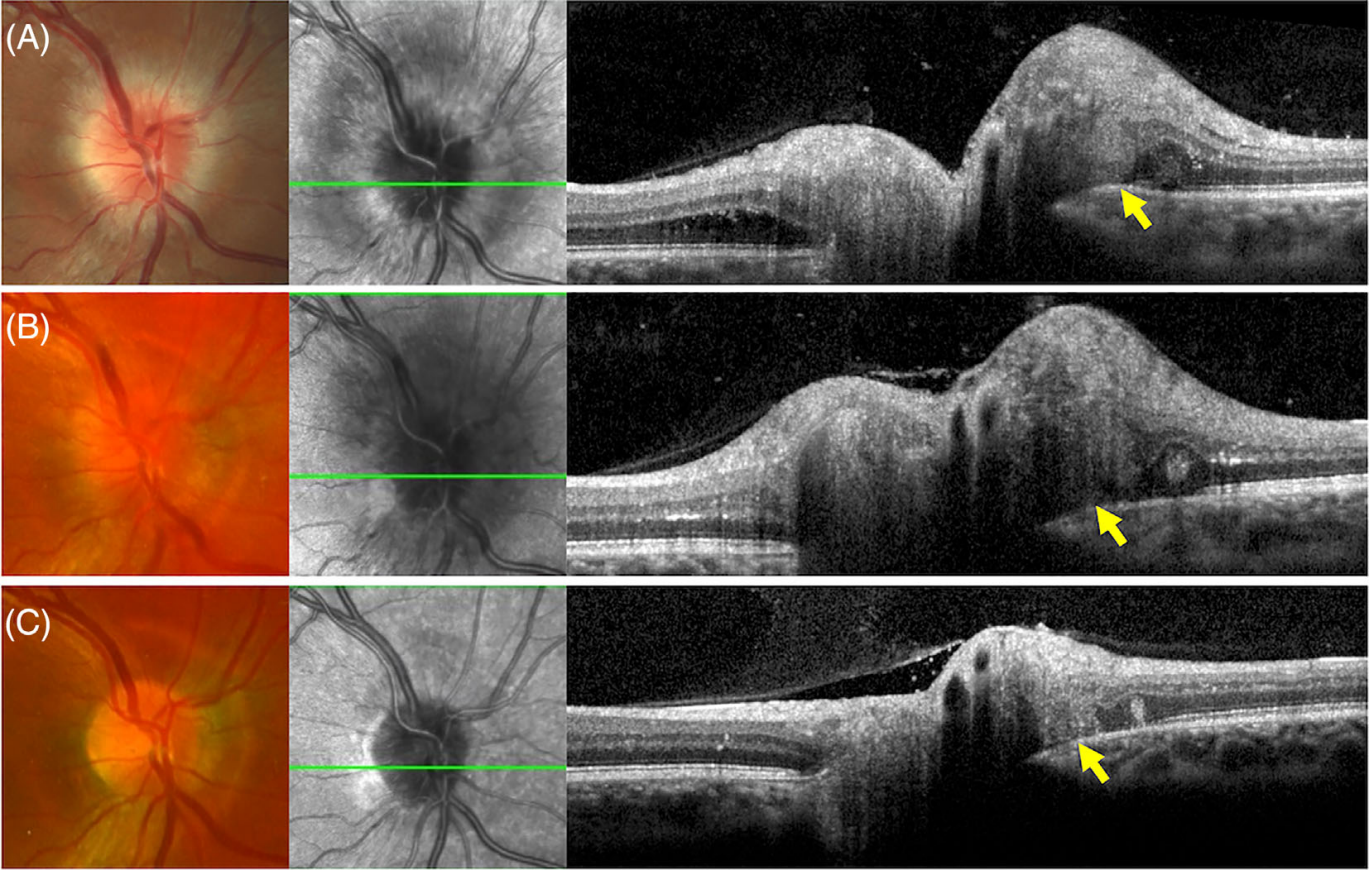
A 28‐year‐old male presented with a right subtle blurry patch of vision accompanied by pain on eye movement. Disc oedema with peripapillary intraretinal fluid and nerve fibre swelling was noted (A). A peripapillary hyperreflective ovoid mass‐like structure became more prominent 2 weeks later (B). By 3 months, vision had recovered with resolution of the disc oedema but persistence of the PHOMS (C). Throughout the course of this episode, visual acuity remained 6/6 and magnetic resonance imaging pre and post gadolinium showed no abnormal signal or enhancing mass along the right optic nerve sheath complex. There was no evidence of intracranial demyelination.
